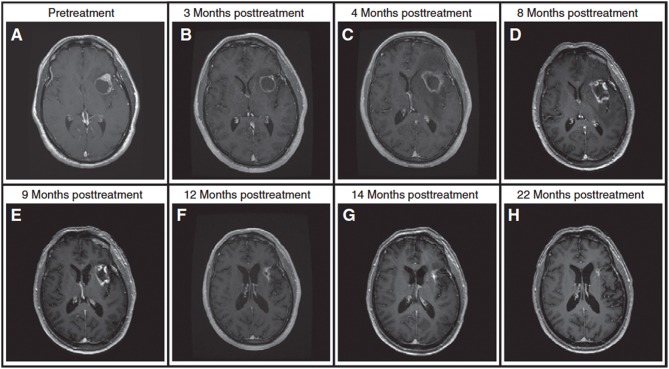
A patient with brain metastasis treated with SRS and presenting with an enlarging enhancing mass after treatment. (A) Pre-treatment. (B) An enlarging, enhancing mass at the 3-month follow up scan, (C) lesion is larger at 4-month follow up. Standard MRI at 3-month and 4-month follow up scans is unable to determine if the lesion is tumor progression or radiation necrosis (lesion is near the language center prohibiting its complete resection). (D) A trial of steroids leads to a slight reduction in the enhancing mass but a significant decrease in the surrounding FLAIR at 8-month follow up. (E) Continuing steroids leads to further reduction in enhancing lesion at 9-month follow up. (F–H) Follow-up MRIs at 12 to 22 months post-treatment scans demonstrate significant decrease in tumor size, rendering a diagnosis of radiation necrosis (the diagnosis is also confirmed with DWI and T_2w_ FLAIR). In two occasions during the period of uncertainly, the patient was admitted to hospital with neurological symptoms. For this patient, it took longer than 9 months to render a diagnosis, demonstrating the challenges faced in clinic in differentiating radiation necrosis from tumor progression. Reproduced, with permission from Mehrabian et al. (72).
